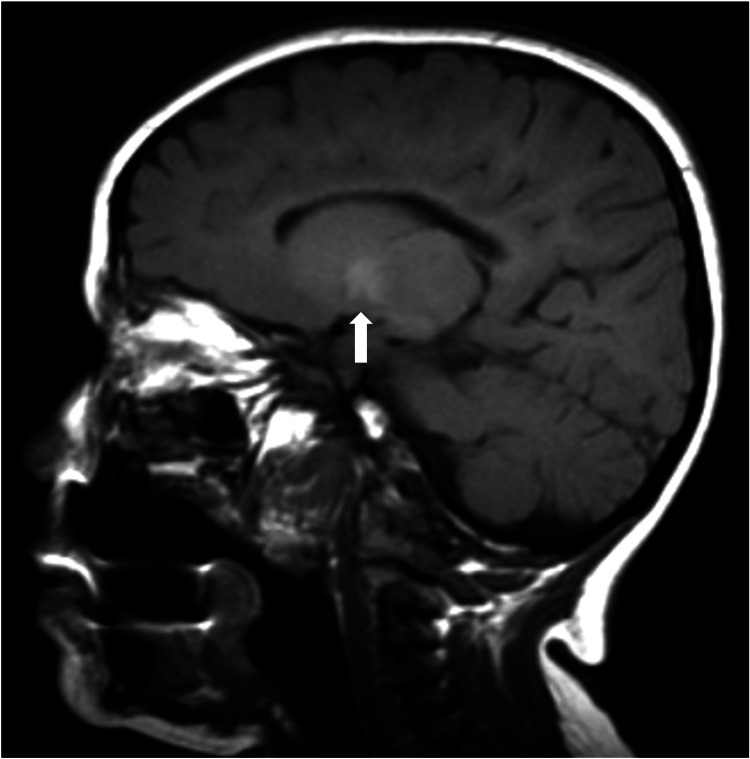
Sagittal T1 Weighted Imaging Sagittal T1-weighted image shows relative hyperintense signal within the globus pallidus with a hypointense streak corresponding to the medial medullary lamina (white arrow).
